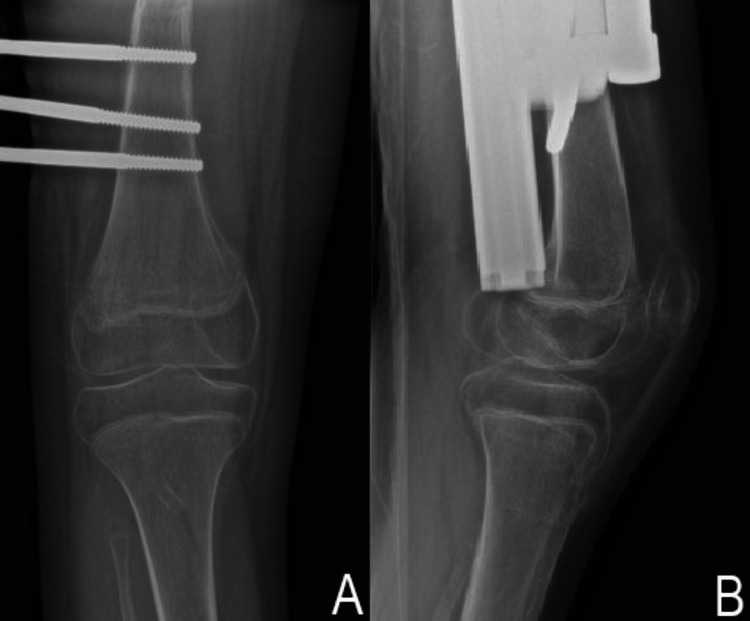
Repeat radiographs of the right knee Anteroposterior (AP) (A) and lateral (B) radiographs of the right knee showing callus formation surrounding the fracture site.
